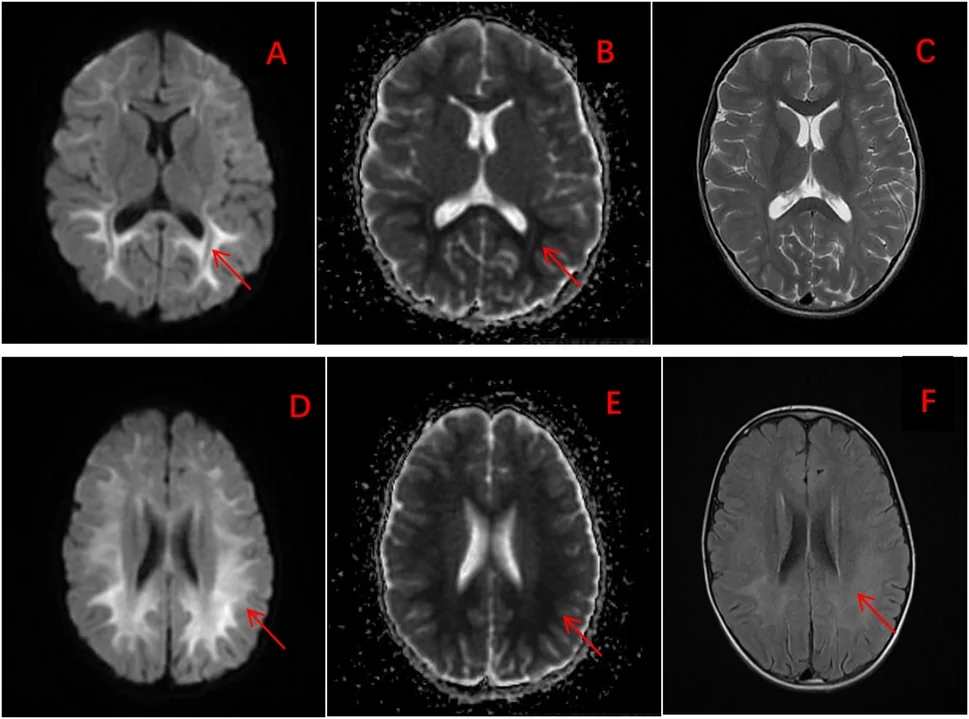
Brain MRI results of children with hypersarcosinemia (February 12, 2025, half a month after discharge).A and D: Large patches of DWI hyperintense signal in the subcortical white matter of the bilateral cerebral hemispheres (arrows). B and E: Large patches of subcortical white matter in the bilateral cerebral hemispheres showed hypointense on ADC maps (arrows). C: T2-weighted images showed no abnormalities. F: Slight FLAIR hyperintensity in the white matter of the posterior horn of the bilateral ventricles (arrows). MRI, magnetic resonance imaging. DWI, diffusion-weighted imaging. ADC, apparent diffusion coefficient. FLAIR, fluid-attenuated inversion recovery.
